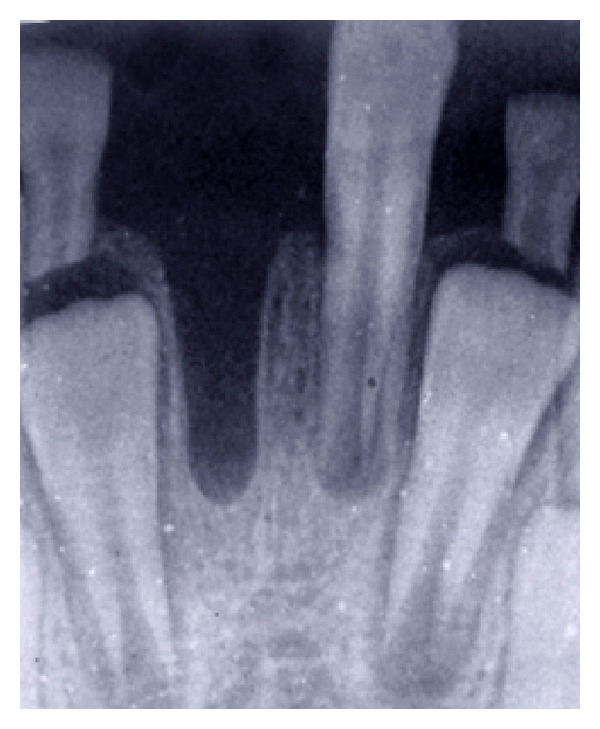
Preoperative radiograph showing the intact socket of right lower central incisor.
